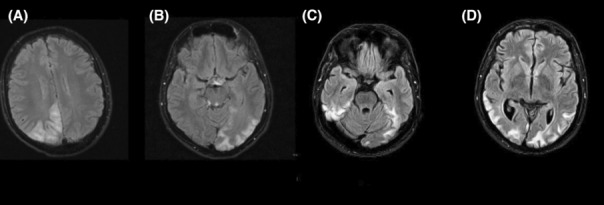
(A) Fluid attenuated inversion recovery (FLAIR) images in the year 2013, when the patient was 27 years old, showing ischemic lesions in the right occipital lobe and posterior parietal regions. (B) FLAIR images in 2014, when the patient was 28 years old, showing left occipital and posterior parietal lobe ischemia. (C, D) In the year 2020, as the patient was 34 years old, FLAIR images revealing bilateral ischemic lesions on the occipitoparietal regions and generalized brain atrophy.
